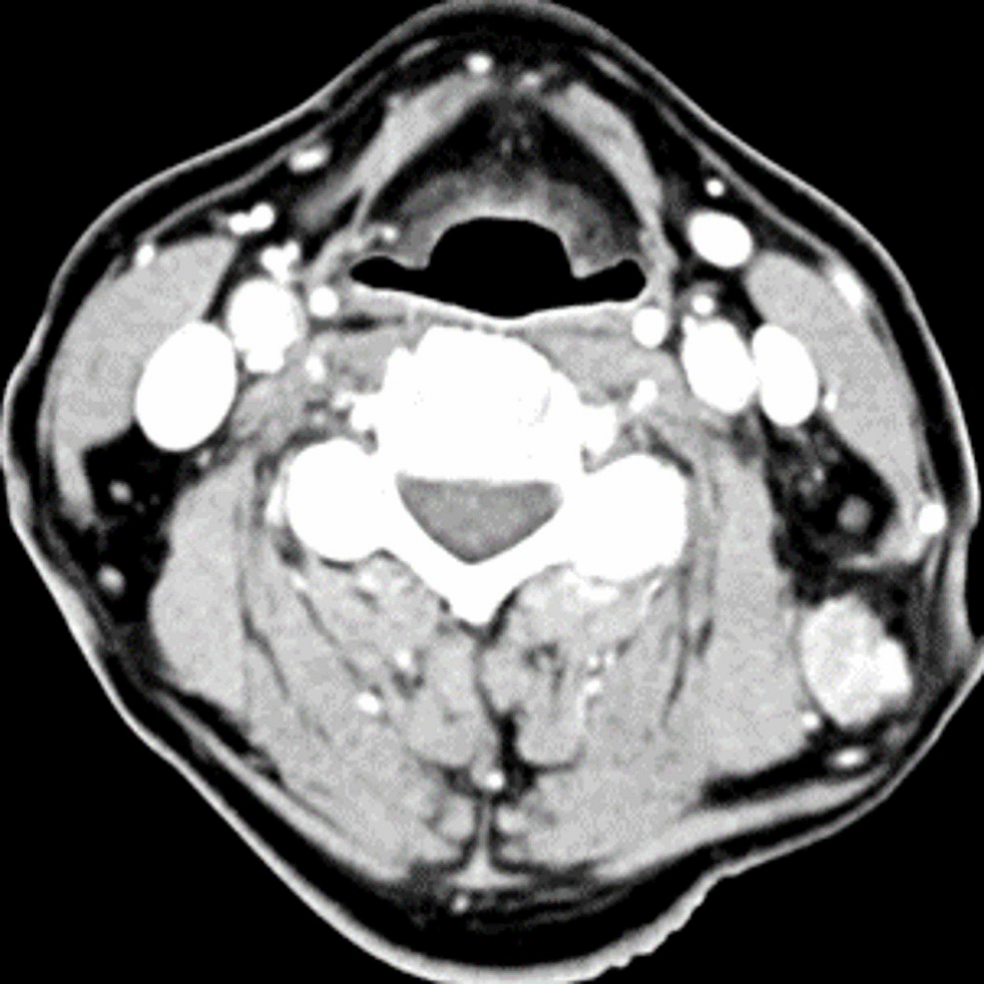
CT head and neck axial view thin sections demonstrating nodal metastasis with pathologically enlarged level 3 and 5 lymph nodes, that is, the posterior upper jugular and upper posterior triangle lymph nodes CT: computed tomography
